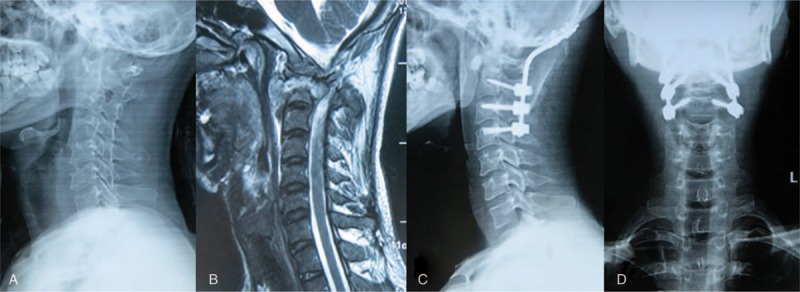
A 27-year-old man was diagnosed as having upper cervical tuberculous spondylitis after a 3 months history of severe cervical pain. X-ray and MRI showed the destruction of vertebral bodies of C0–C2 (A–B), final follow-up radiographs showed good bone fusion (C–D). MRI = magnetic resonance imaging.
